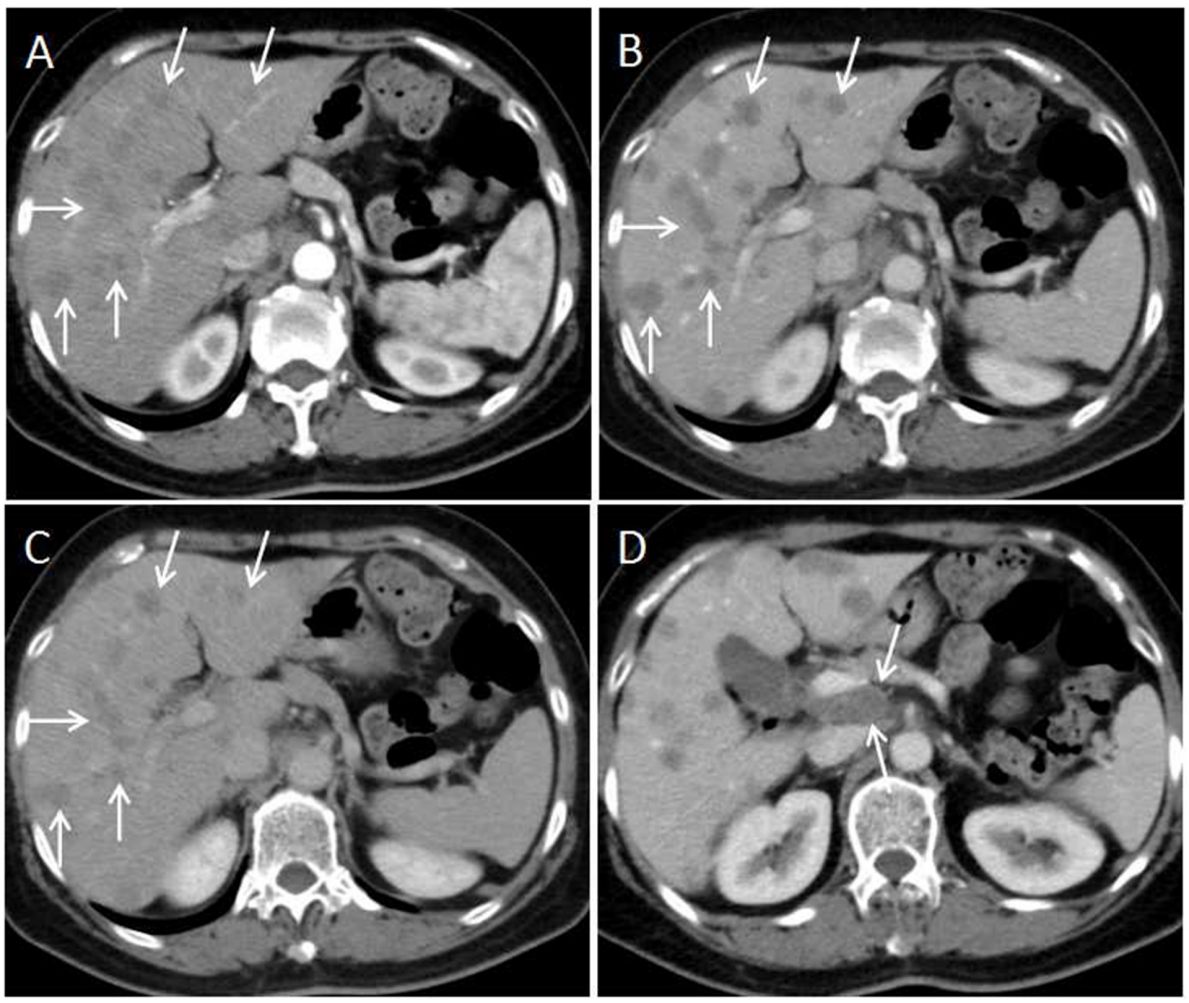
63-year-old woman with moderately differentiated colon adenocarcinoma (A) Multiple hypo enhanced liver metastases are presented on hepatic arterial phase CT image(arrows). (B and C) On the portal venous phase and equilibrium phase images, the lesions keep hypo enhancement that meets the plateau enhancement pattern(arrows). (D) Axial CT image on the portal venous phase presents lymphadenopathy between the portal vein and venae cava inferior (arrows).
